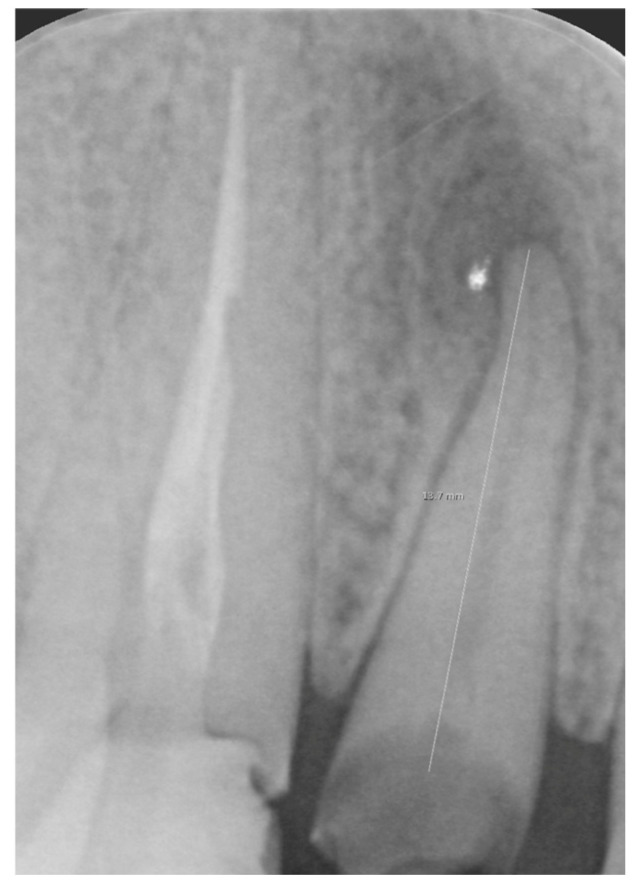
Intraoral x-ray of a tooth with a destructive carious lesion.
